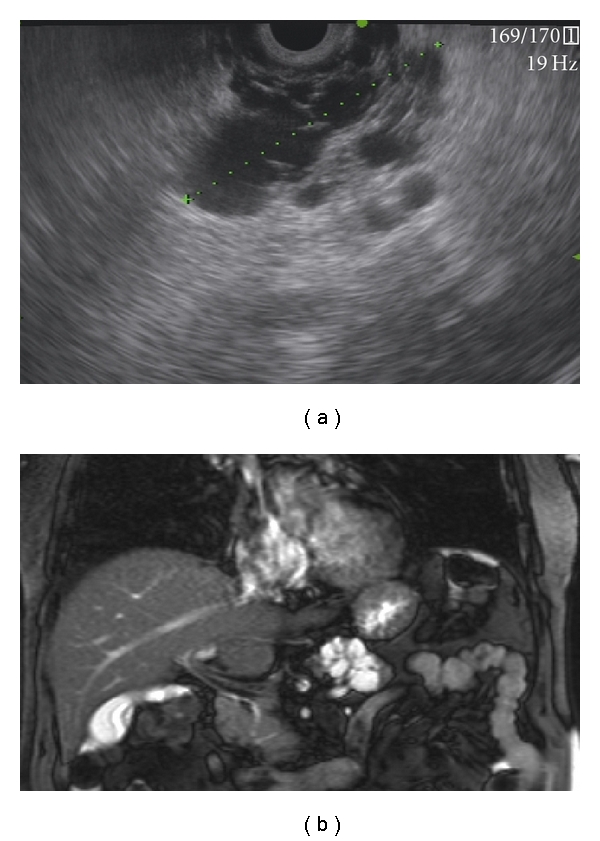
(a) EUS image of a serous cystadenoma in the head of the pancreas. (b) MRI image of a serous cystadenoma in the head of the pancreas.
